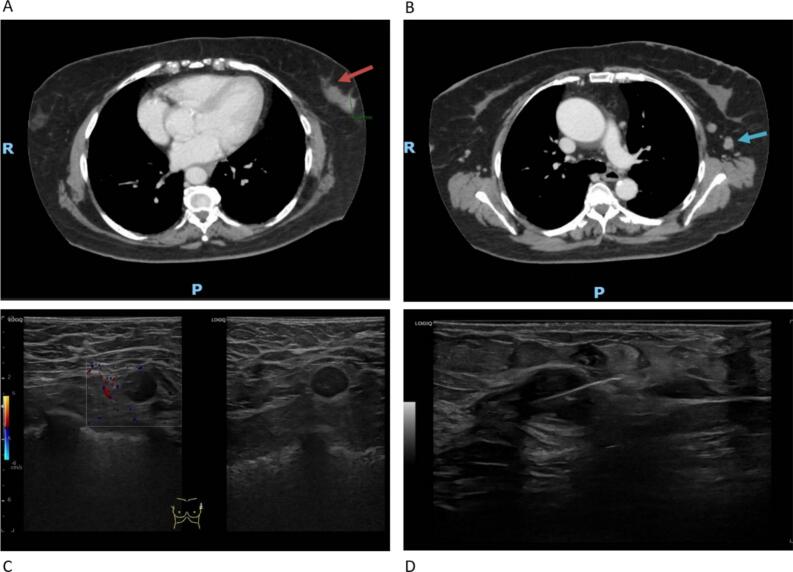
A. Axial contrast-enhanced CT (portal venous phase) shows an enhancing, slightly spiculated mass in the left breast (red arrow). 2B – At a higher axial level, two round left axillary lymphadenopathies with cortical thickening are identified (blue arrow). 2C – Ultrasound image shows the left axillary lymphadenopathies. 2D – Ultrasound-guided needle biopsy of the left breast lesion. (For interpretation of the references to colour in this figure legend, the reader is referred to the web version of this article.)
